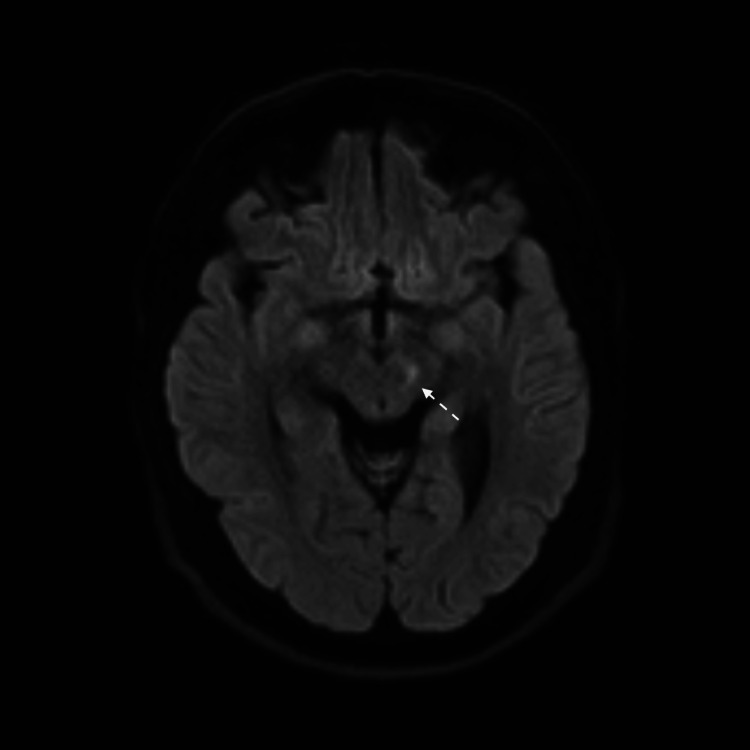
MRI brain DWI view shows left midbrain cerebral peduncle infarction.
